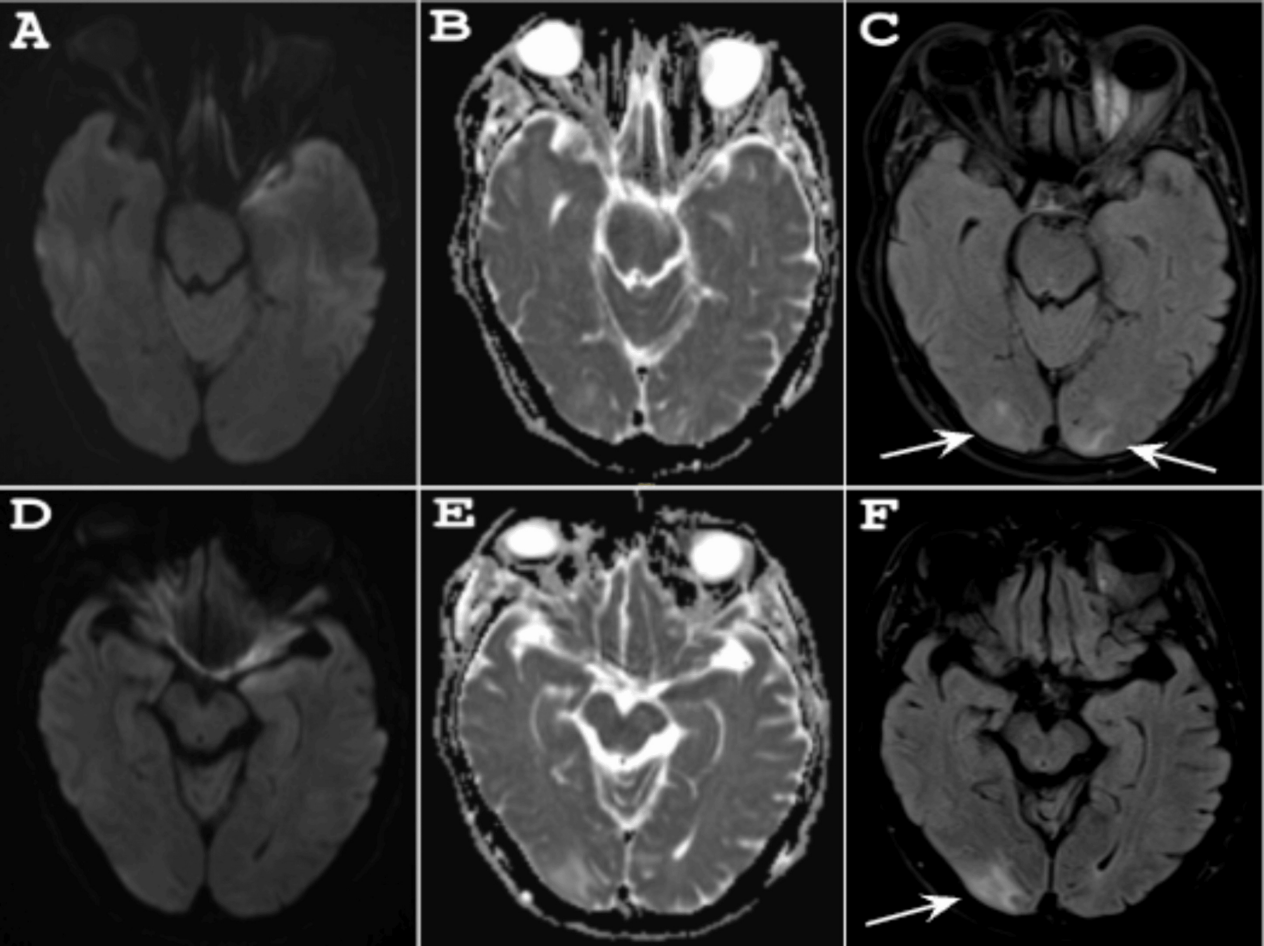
MRI of the brain pre-treatment (A, B, D, E) Axial DWI and ADC showing no evidence of diffusion restriction, while (C, F) FLAIR demonstrates hyperintensities of the right parietal and bilateral occipital lobes suggestive of vasogenic edema. MRI: magnetic resonance imaging; DWI: diffusion-weighted imaging; ADC: apparent diffusion coefficient; FLAIR: fluid-attenuated inversion recovery
